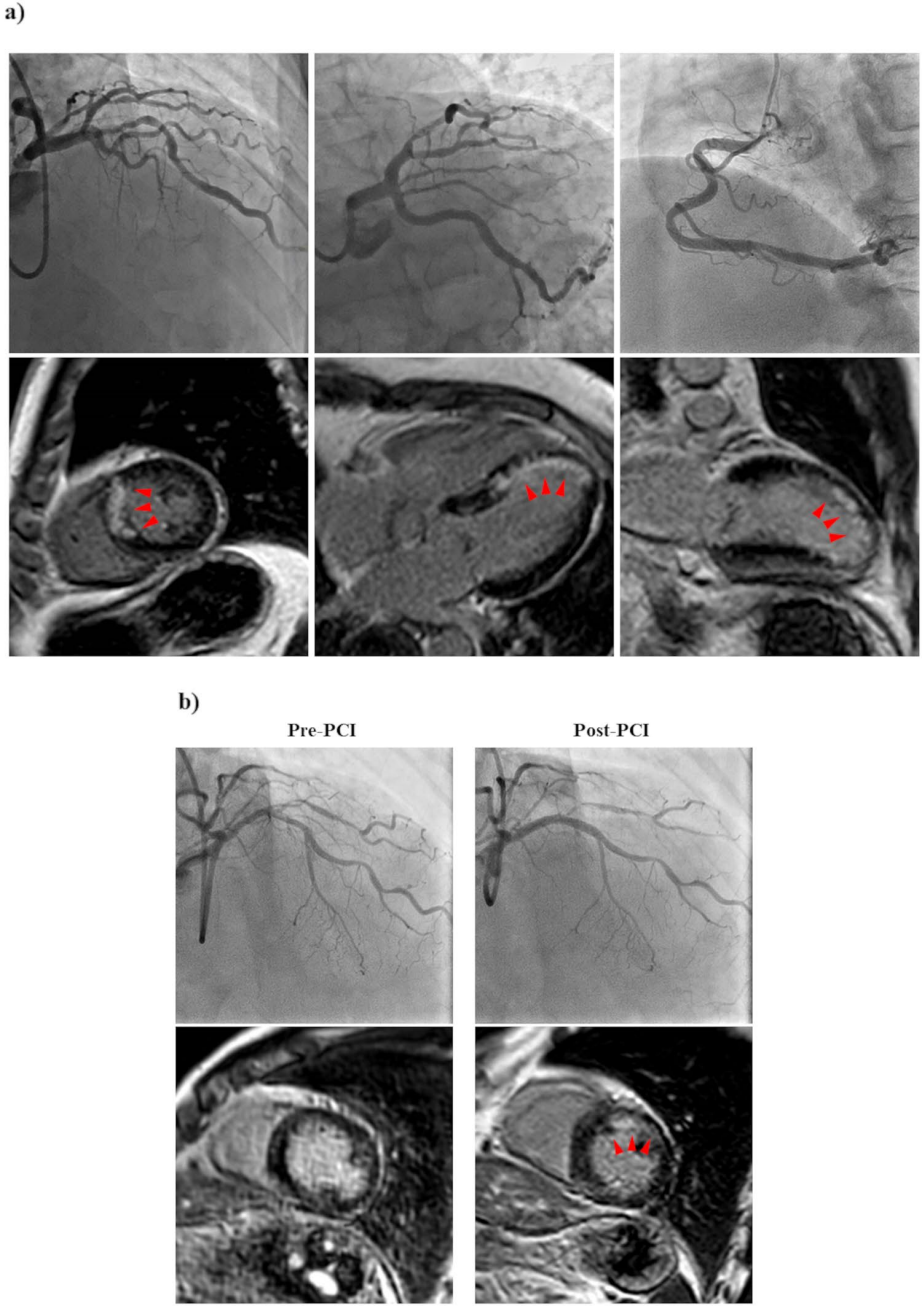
Representative cardiac magnetic resonance images and angiographies (a) of a patient with unrecognized myocardial infarction; (b) of a patient with periprocedural new occurrence of LGE after PCI.
